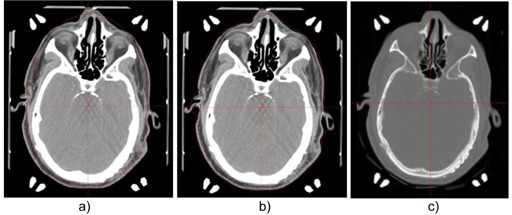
A sample transverse CT slice with the skull contours from the manual skull definition method (a) and the imaging skull definition method (b). The electron density map for the slice is shown in (c).
